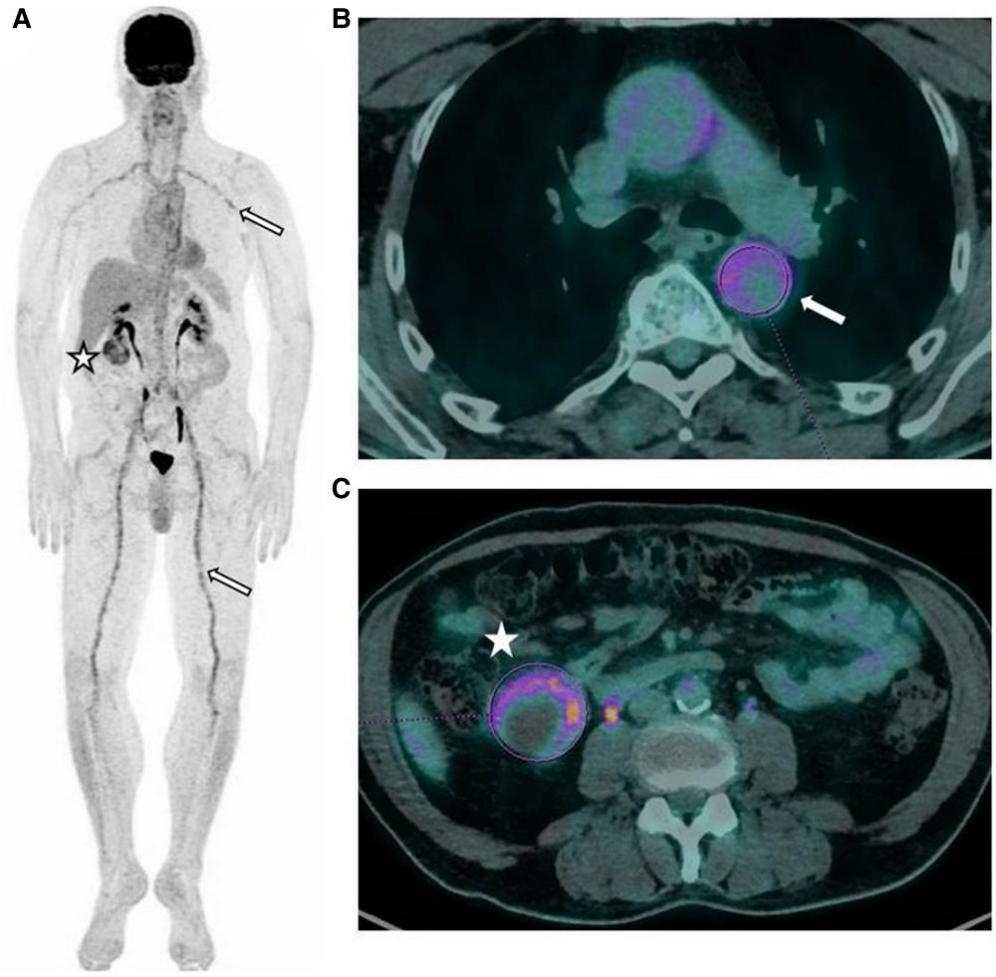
Large vessel arteritis diagnosis using positron emission tomography/computed tomography. Initial fluorodeoxyglucose-positron emission tomography scan maximum intensity projection (*A*) and fused positron emission tomography/computed tomography coronal views (*B*, *C*) revealed diffuse hypermetabolism of large vessels, consistent with vasculitis (arrows). Pathological fluorodeoxyglucose uptake was also observed in a heterogeneous right renal mass (star).
